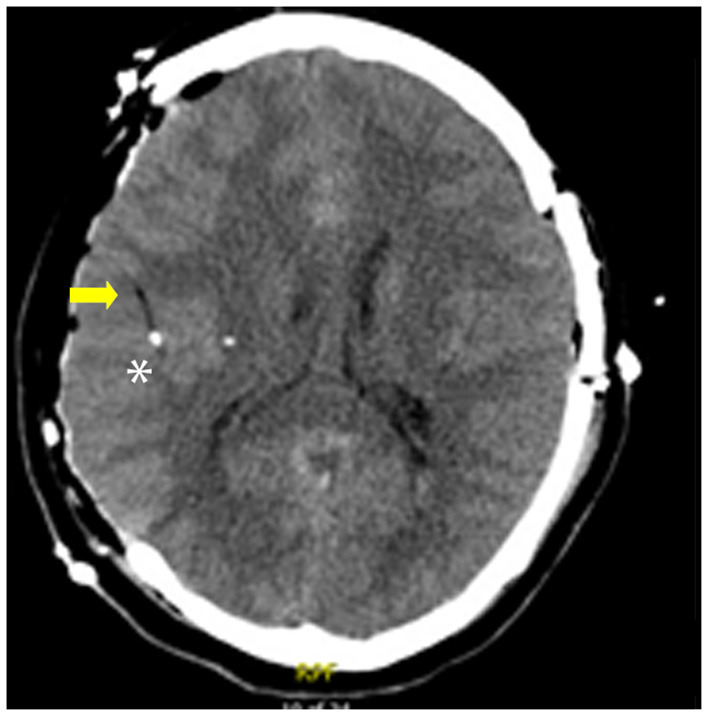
Example of the gold probe tip on CT a patient who underwent hemicraniectomy. Note the hypodense linear catheter (arrow), and hyperdense gold filament tip (*).
